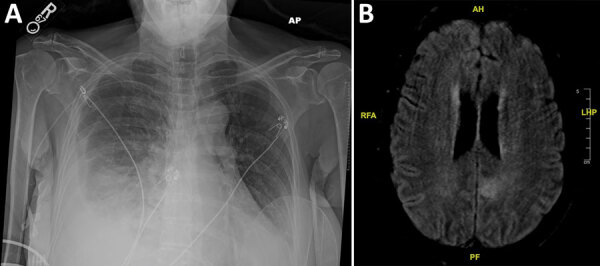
Imaging studies from a 65-year-old woman with multiple myeloma undergoing chimeric antigen receptor T-cell therapy who was admitted to the hospital for worsening foot and ankle pain, California, USA. A) Chest radiograph image, showing a moderate right-sided pleural effusion and adjacent pulmonary opacities indicative of pneumonia. B) Magnetic resonance imaging of the patient’s brain, showing the infarct involving the left medial parietal lobe.
